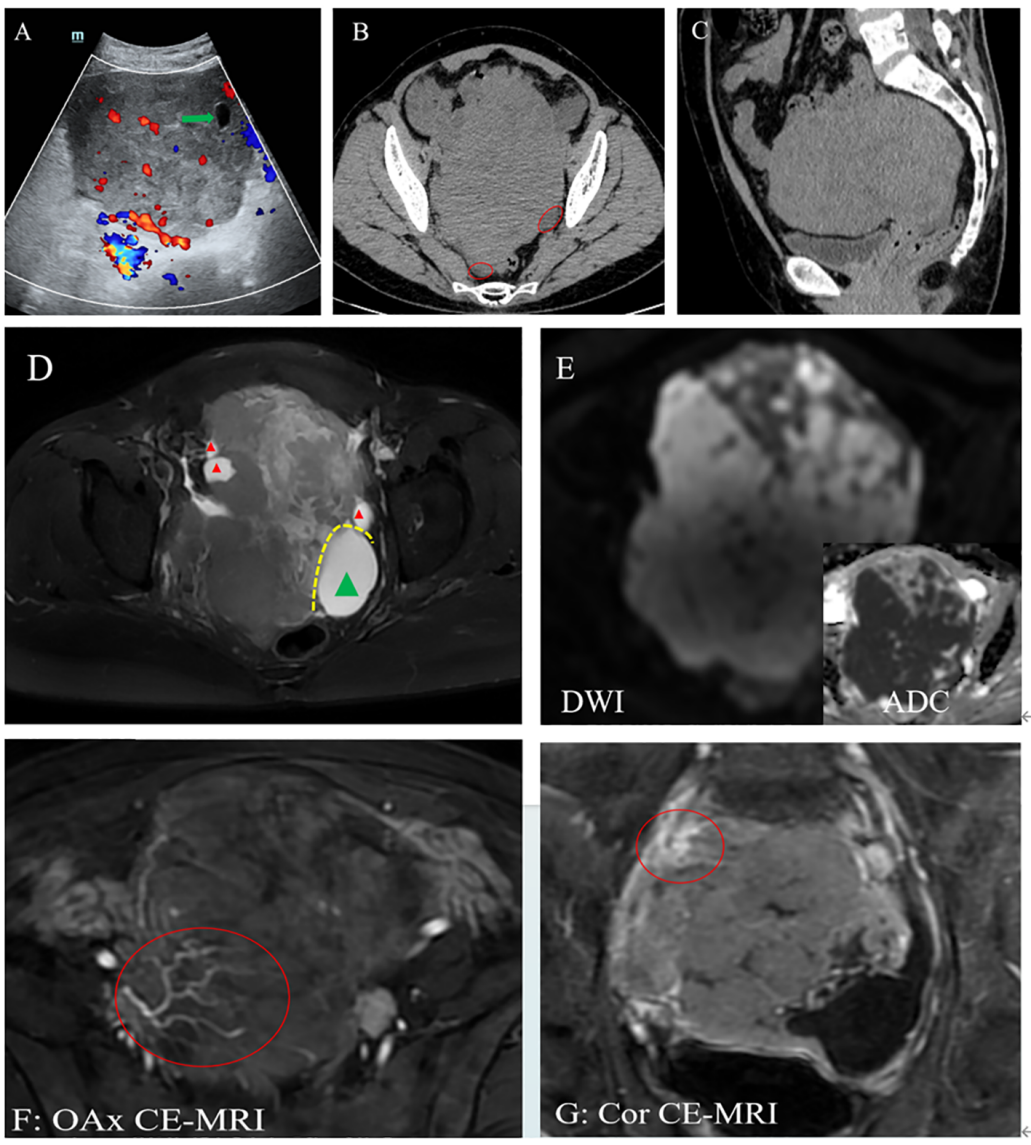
(A) Ultrasound (US): A hypoechoic lesion in the pelvis with a clear boundary and a regular shape, with blood flow signal. A cystic area was at the edge of the lesion (*green arrow*). (B, C) Computed tomography (CT): A heterogeneous hypodense mass with ascites (*red circle*). The CT value was approximately 40 HU. The adjacent bowels and urinary bladder were pushed. (D–G) Magnetic resonance imaging (MRI): Plain scan showing the right ovary as enlarged with multiple peripheral follicles seen at the edge on T2-weighted imaging (T2WI) (*red triangle*). (D) Left ovary showing cystic enlargement (*green triangle*). These two ovaries are in contact with each other due to enlargement. (E) On diffusion-weighted imaging (DWI), the mass presented as a heterogeneous high signal, and its solid component had a low apparent diffusion coefficient (ADC) value. (F, G) Enhanced image showing vascular floatation sign (F) and the sandwich sign (G), exhibiting that normal blood vessels walked within the tumor and the right ovarian vein was encased by the tumor, respectively.
